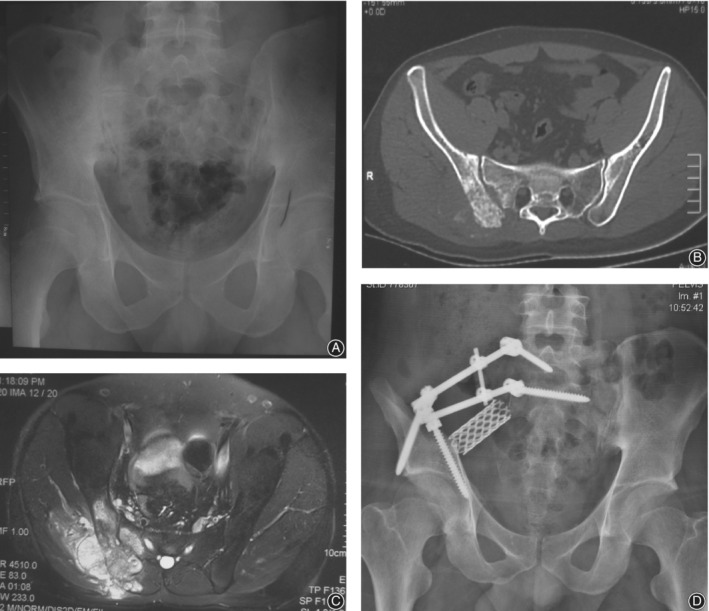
Male, 27 years old, right iliac sacral osteosarcoma. (A) Positive pelvic X‐ray showed sclerotic bone destruction of the right posterior iliac and sacrum before operation. (B) Preoperative CT showed bone destruction and soft tissue mass formation in the posterior part of the right iliac bone, which invaded the right sacral wing. (C) Preoperative MRI showed that the tumors invaded the sacroiliac joint and the right sacral wing through the interosseous ligament. Soft tissue mass invaded the gluteus maximus and gluteus medius muscle. (D) Positive pelvic X‐ray films 1.5 years after operation showed good internal fixation and titanium mesh position without signs of loosening or rupture.
